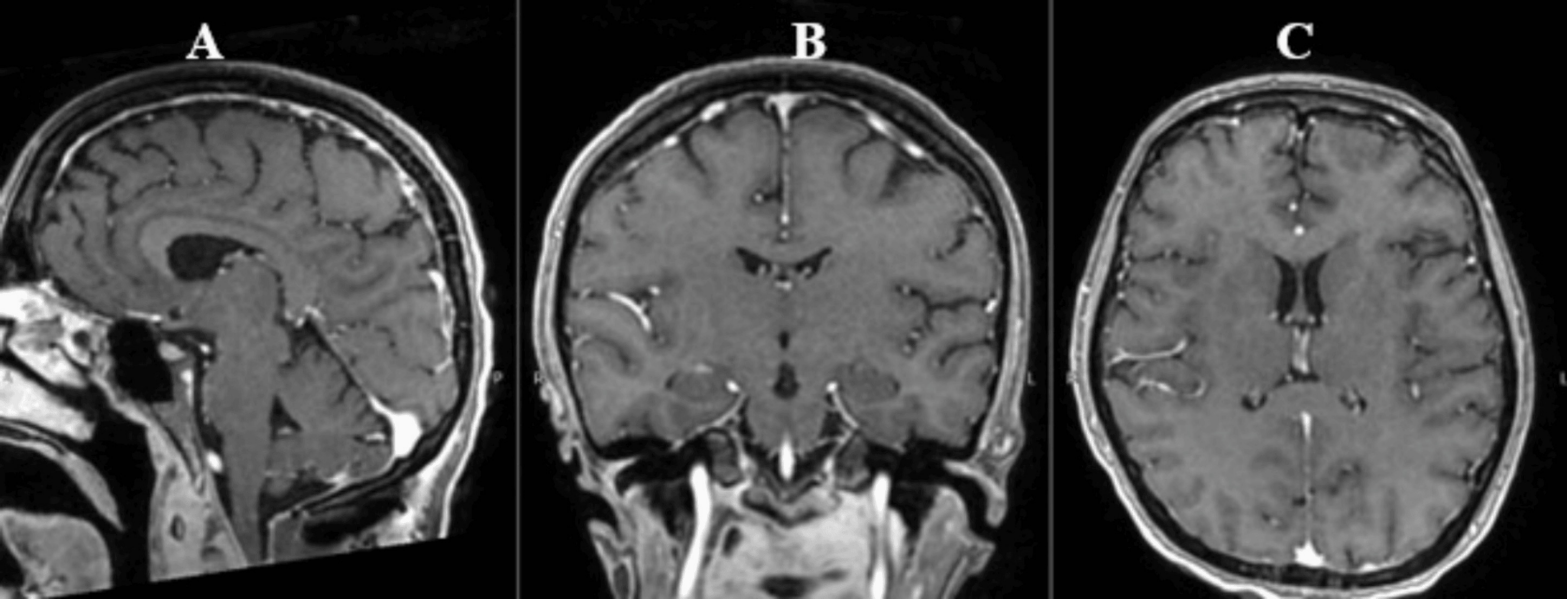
T1 + gadolinium MRI A. Sagittal view B. Coronal view C. Axial view demonstrates the absence of coccidioidomycosis dissemination at the brain level.
